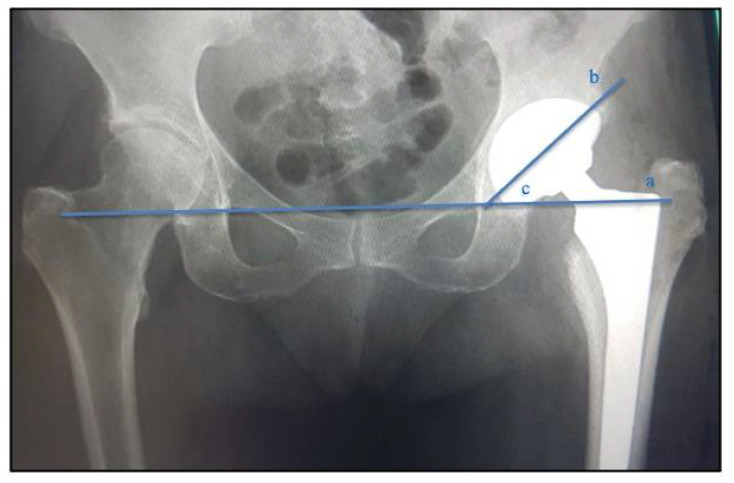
Acetabular component positioning angle measurement. (a)—line that touches the ischial tuberosities; (b)—line through the axis of the largest diameter formed by the projection of the metallic ring on the radiograph; (c)—acetabular angle.
